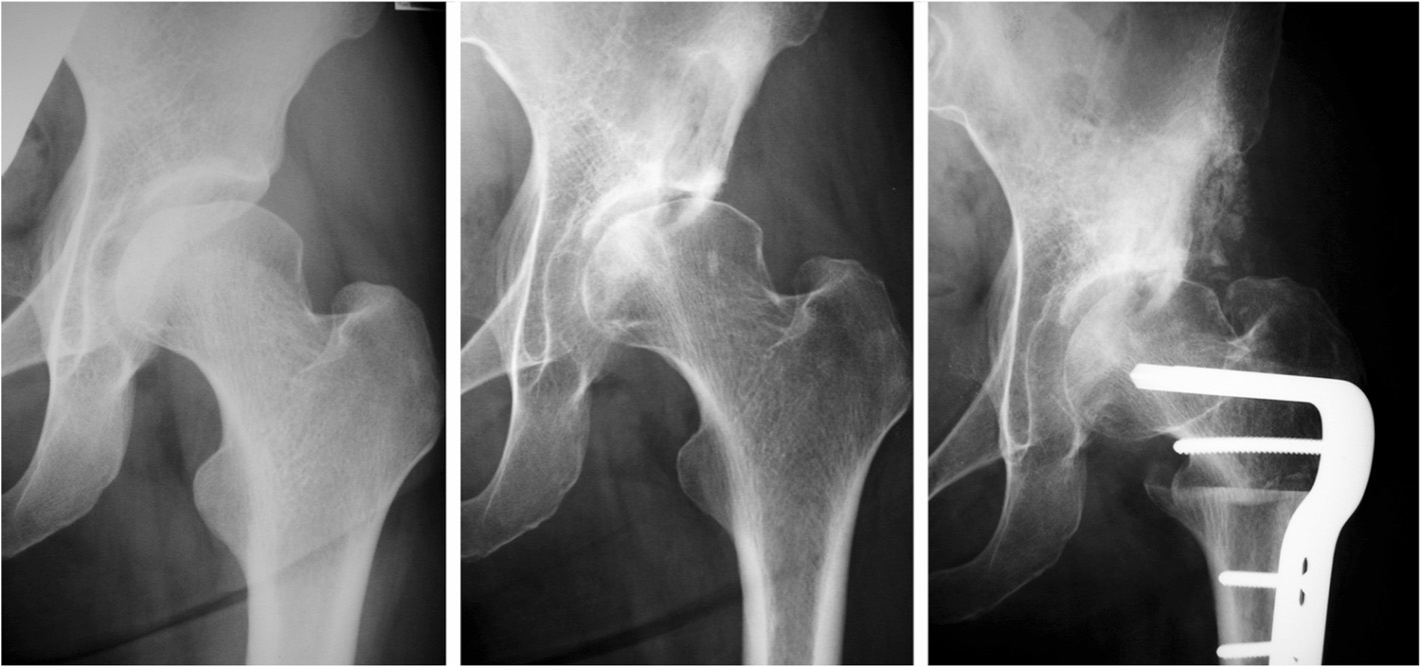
Case that developed arthritic changes (non-dysplastic, positive crossover sign). *Left*: radiograph before the follow-up (age 34 years old, female); the patient underwent Chiari osteotomy on the contralateral side for developmental dysplasia. *Middle*: radiograph from 16 years after the beginning of the follow-up (age 50 years old); the joint space was slightly narrower, and a bone cyst was noted on the acetabular side. *Right*: radiograph after the end of the follow-up (age 51 years old); the patient complained of severe pain in the hip. Therefore, she underwent femoral subtrochanteric varus osteotomy and allogenic bone grafting into the bone cyst to prevent collapse.
